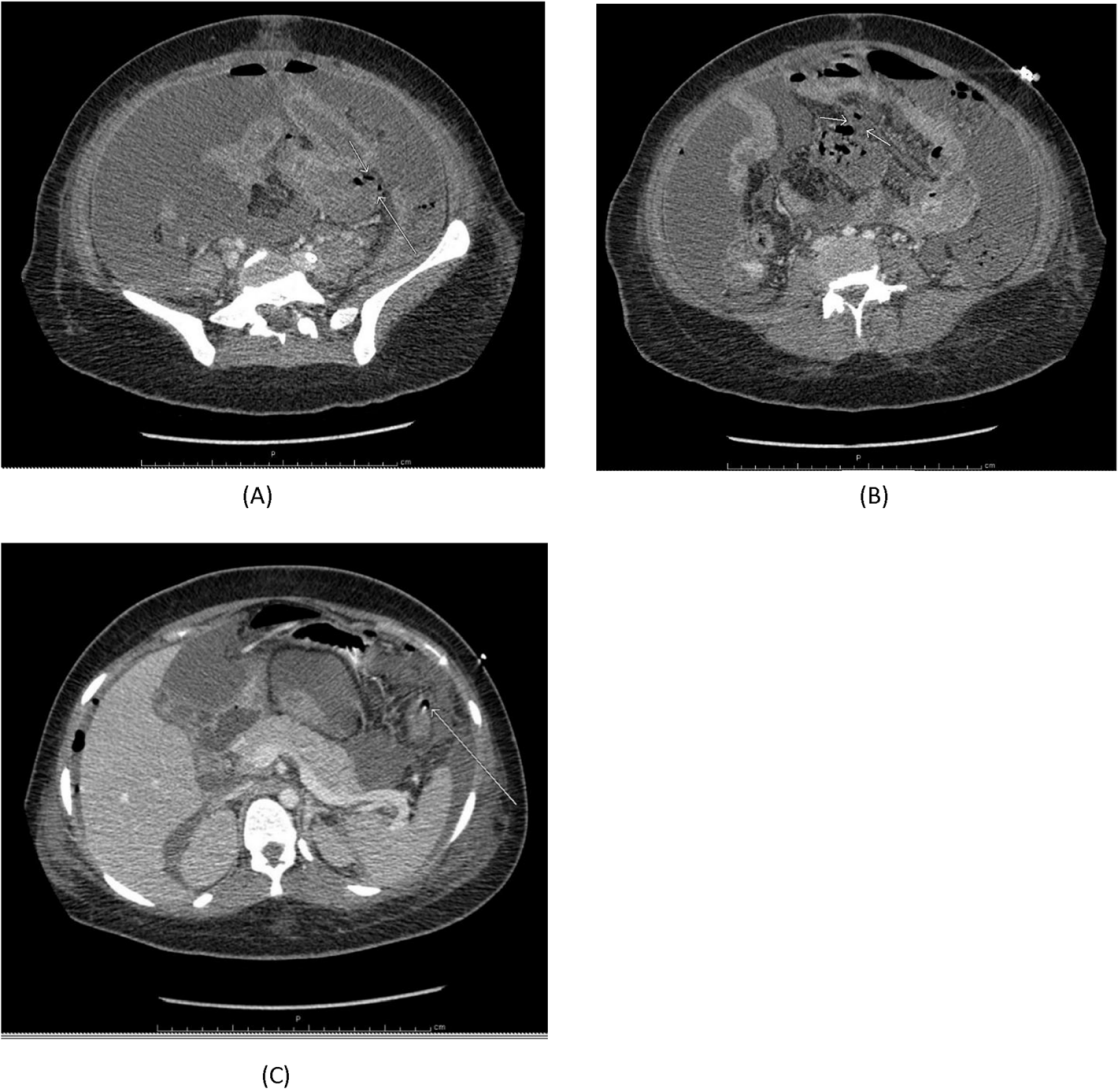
Contrast-enhanced computerized tomography (CT) scan of the abdomen revealed moderate pneumoperitoneum with intraperitoneal fluid. a, b Many sites of disrupted jejunal wall with mottled air from bowel lumen into nearby mesentery was observed, which is consistent with jejunal perforation. c Long segmental bowel wall thickening and marked enhancement along the jejunum, as well as bowel wall disruption at the splenic flexure of the colon, indicated the presence of air outside the colonic lumen
